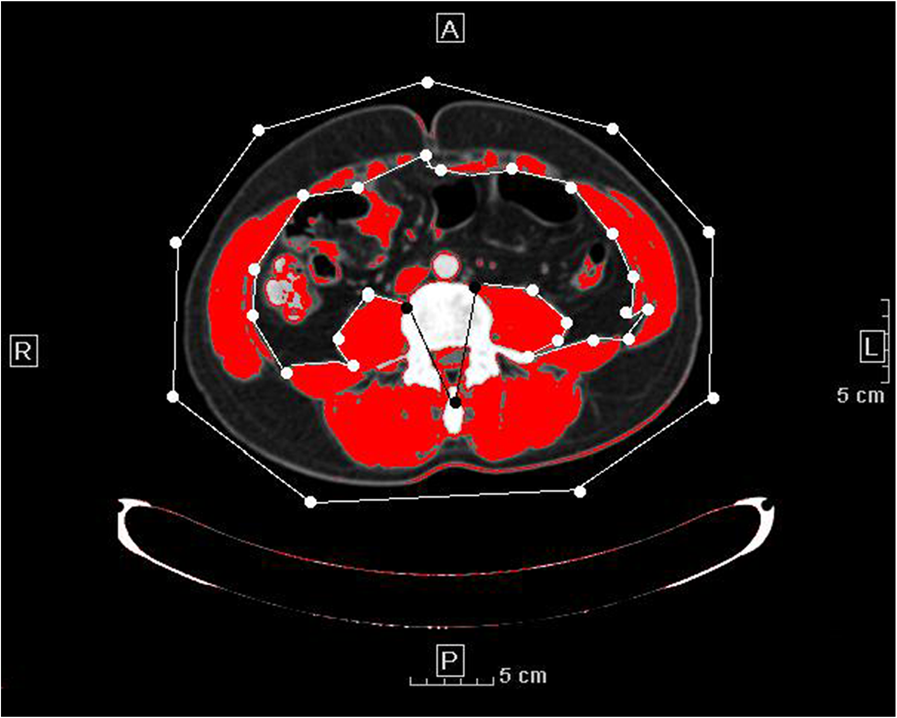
Computed tomography image showing highlighted areas of skeletal muscle mass (red). The protocol for measuring areas of skeletal muscle mass is as follows: after converting image type to 8-bit, set scale and double check the scale with the vertical and horizontal ruler. Draw the two regions of interest (ROIs) including the whole section and visceral only, respectively (in the inner line, we excluded spinal cord because some part of spinal cord is at the same density range). Use the XOR function to set the third ROI of the area excluding visceral. Smooth the section twice to reduce noise of image before setting the predefined threshold and measuring the highlighted area.
